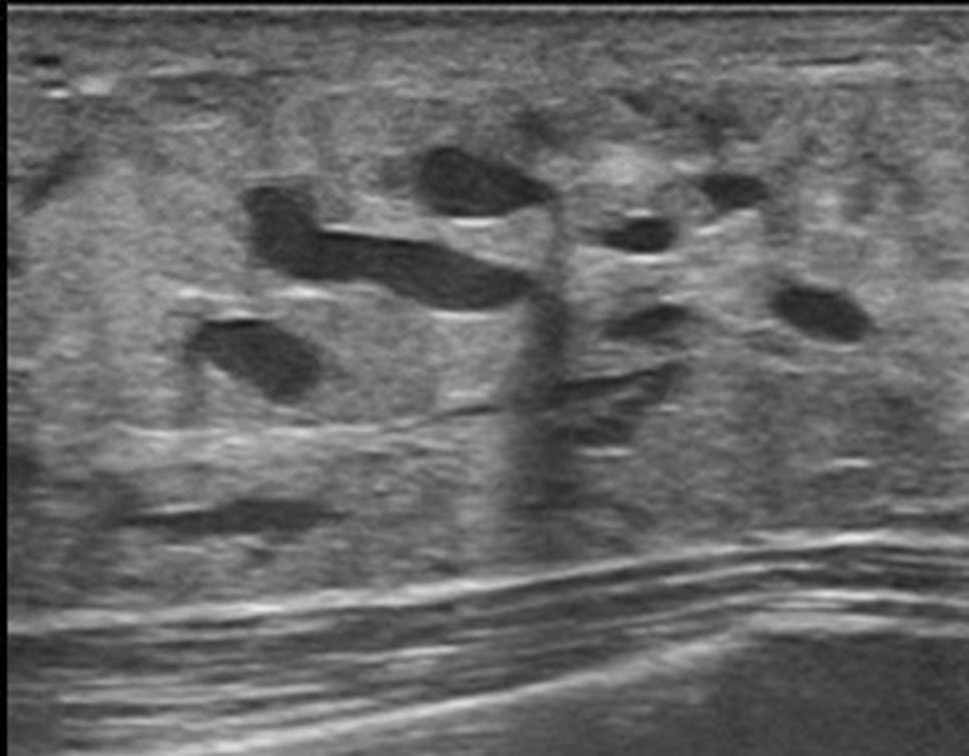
Ultrasound shows duct dilatation, but it is not considered an abnormality based on a finding of duct dilatation alone during late gestation or lactation
